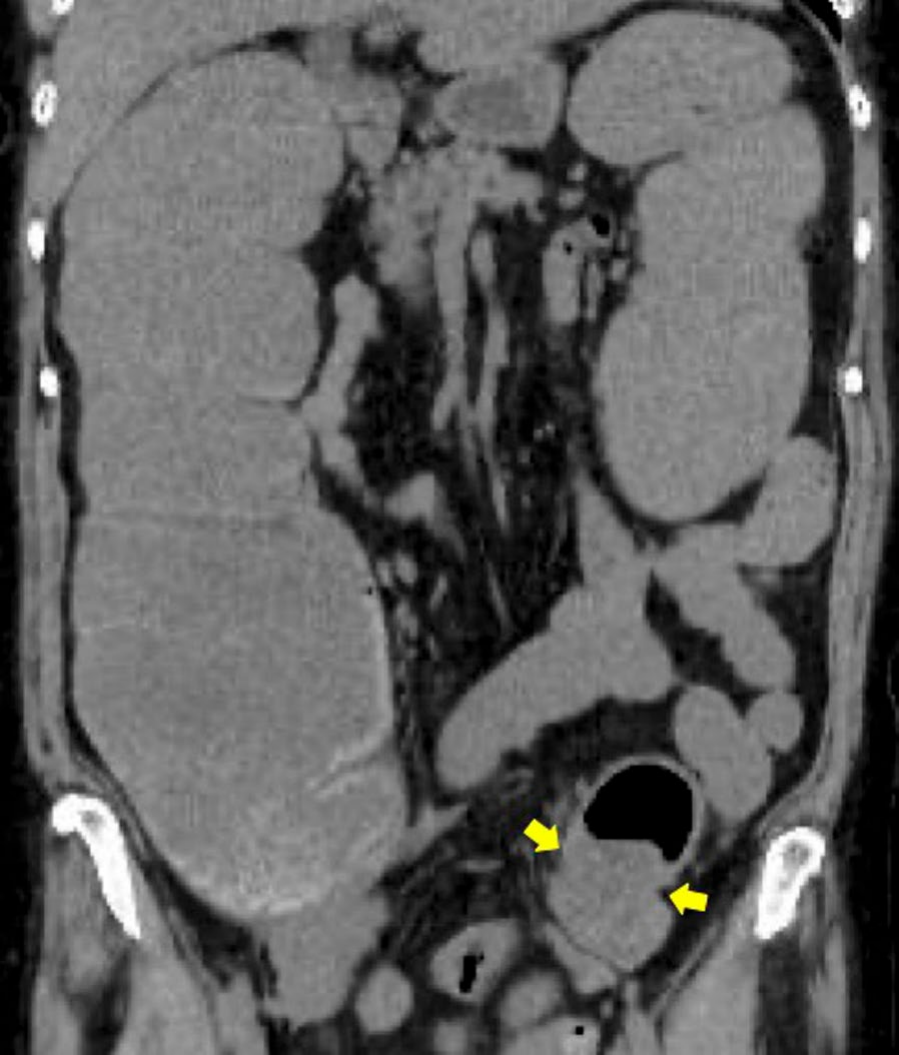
Computed tomography scan obtained before colonic stenting. The scan shows wall thickening in the sigmoid colon (yellow arrows) and dilatation of the colon and small intestine on the oral side of it
